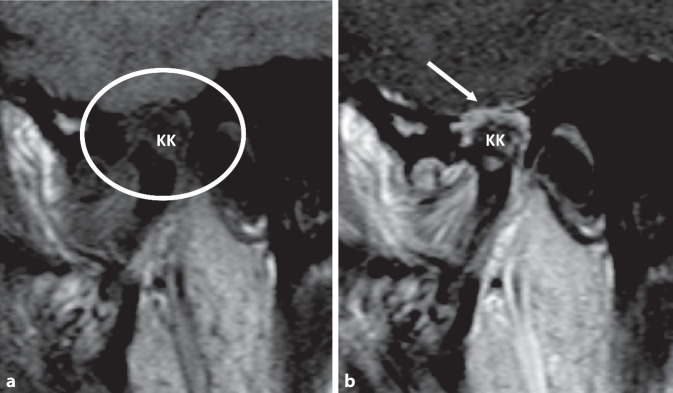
Die nachfolgend durchgeführte CT des Felsenbeins (Abb. 2) zeigte einen kalzifizierenden Prozess, ausgehend vom linken Kiefergelenk. Dieser den Knochen arrosierende Prozess involvierte bildmorphologisch Caput mandibulae, Fossa mandibularis und die mittlere Schädelgrube mit expansiv vorwachsenden Kalzifikationen gegen das Mittelohr und den äußeren Gehörgang. Pathologische Lymphknoten konnten nicht detektiert werden.
